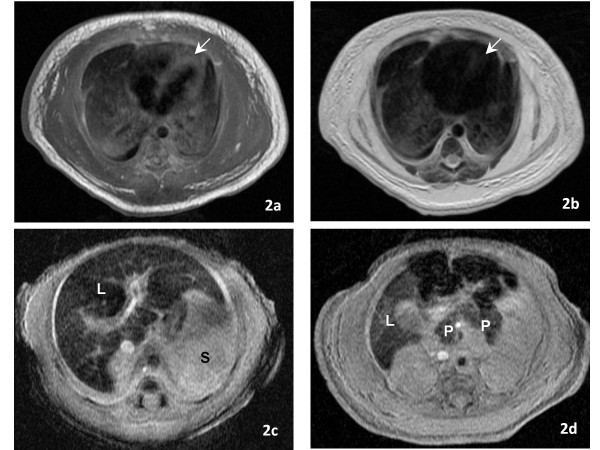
a and b: T1-weighted (1A) and T2-weighted (1B) spin echo axial MR images at the level of the heart show marked drop in myocardial signal intensity (arrow) on T2-weighted image, reflecting myocardial hemosiderin deposition. c and d: Axial T2 STAR gradient echo MR images of the abdomen at the level of the liver and spleen (Fig. 2) and liver and pancreas (Fig. 3) show low signal intensity in the liver (L) and pancreas (P) and normal signal intensity in the spleen (S), reflecting hepatic and pancreatic siderosis.
